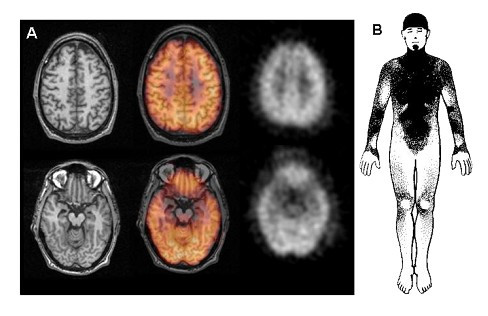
A) Head MRI (left panels) demonstrates cortical atrophy in frontal and temporal lobes. Positron emission tomography (PET) with 2-deoxy-2-fluoro- [^18^F]-D-glucose (FDG) PET (right panels) demonstrates hypometabolism in the same cortical regions. Middle panels show co-registration of MRI and FDG PET studies. B) Thermoregulatory sweating tests in the same patient with PPND. Shaded areas represent sweating over the anterior body surface. Distal anhidrosis is seen.
